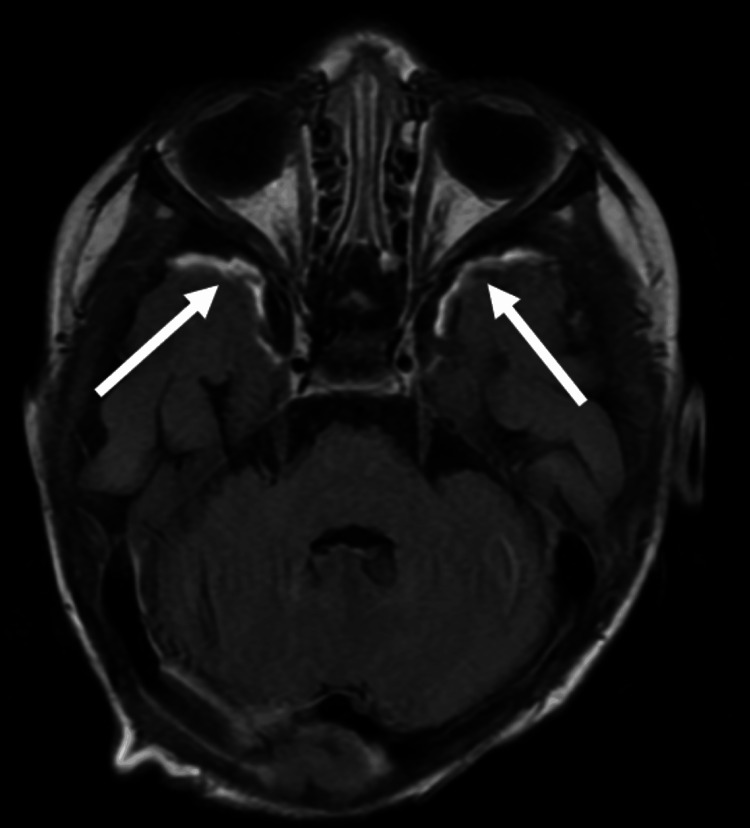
MRI brain, axial view, FLAIR T2 post-contrast, shows the meningeal enhancement in the floor of the anterior cranial fossa bilaterally (arrows). FLAIR: fluid-attenuated inversion recovery
